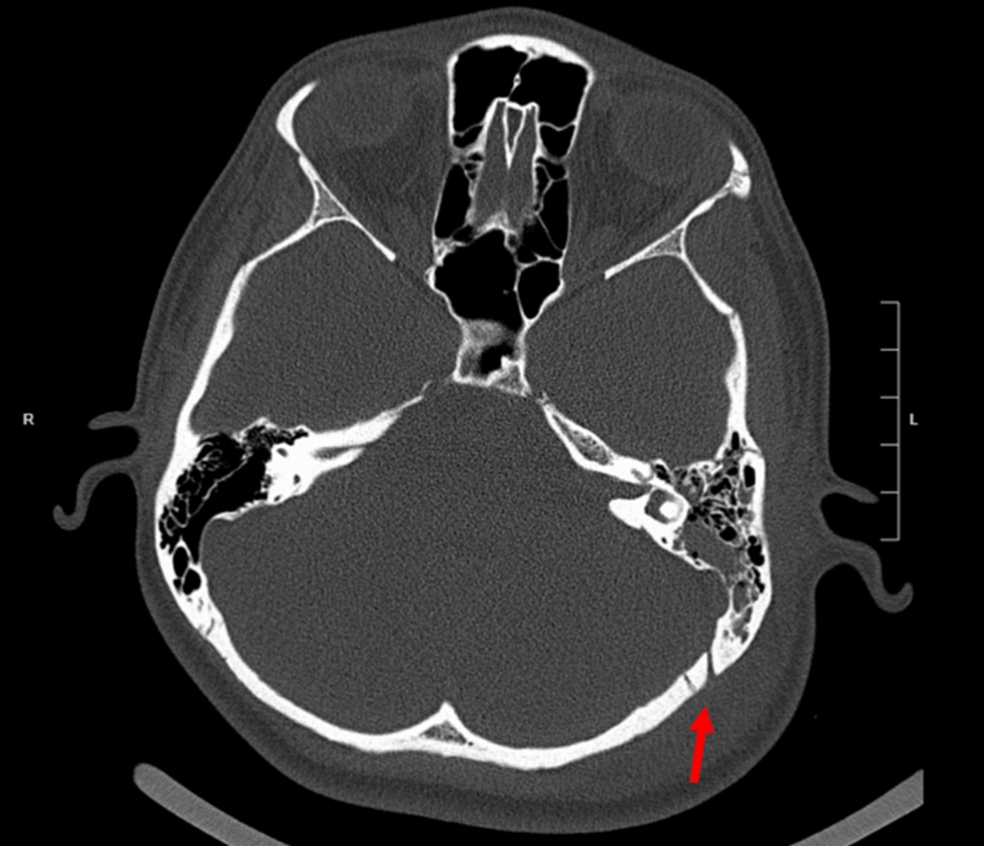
Head CT Primary CT Impression: Left basilar skull fracture communicating with the ipsilateral mastoid air cells.
